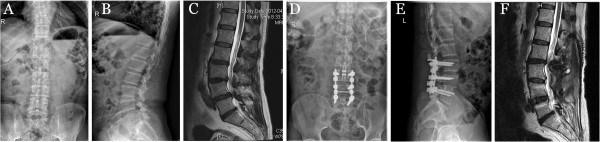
Patient with L4/L5 grade I spondylolisthesis, L3/L4 and L4/L5 protrusion of intervertebral disc, spinal stenosis. (A) Preoperative lumbar radiographs. (B) Preoperative lateral lumbar spine MRI. (C) L3/L4 grade IV intervertebral disc degeneration. (D) ISOBAR TTL internal fixation system, L3/L4 implanted microactuator device, the postoperative lumbar radiograph. (E) Postoperative lumbar lateral film. (F) Lumbar intervertebral disc MRI after 2 years, L3/L4 grade II lumbar intervertebral disc degeneration.
